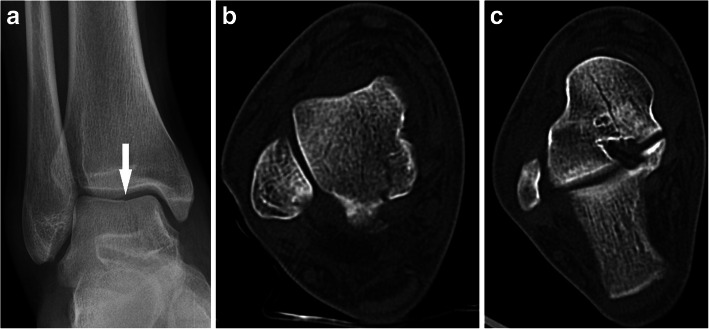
Sagittal nondisplaced fracture of body, neck & head in a 28-year-old man. Radiograph (a), CT sections (b & c)
